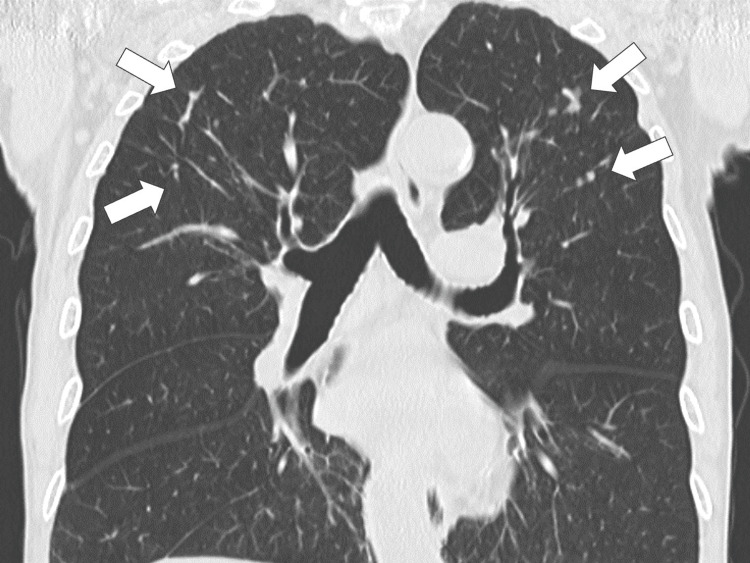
The chest computed tomography revealing predominant nodules in both upper lung fields (white arrows)
